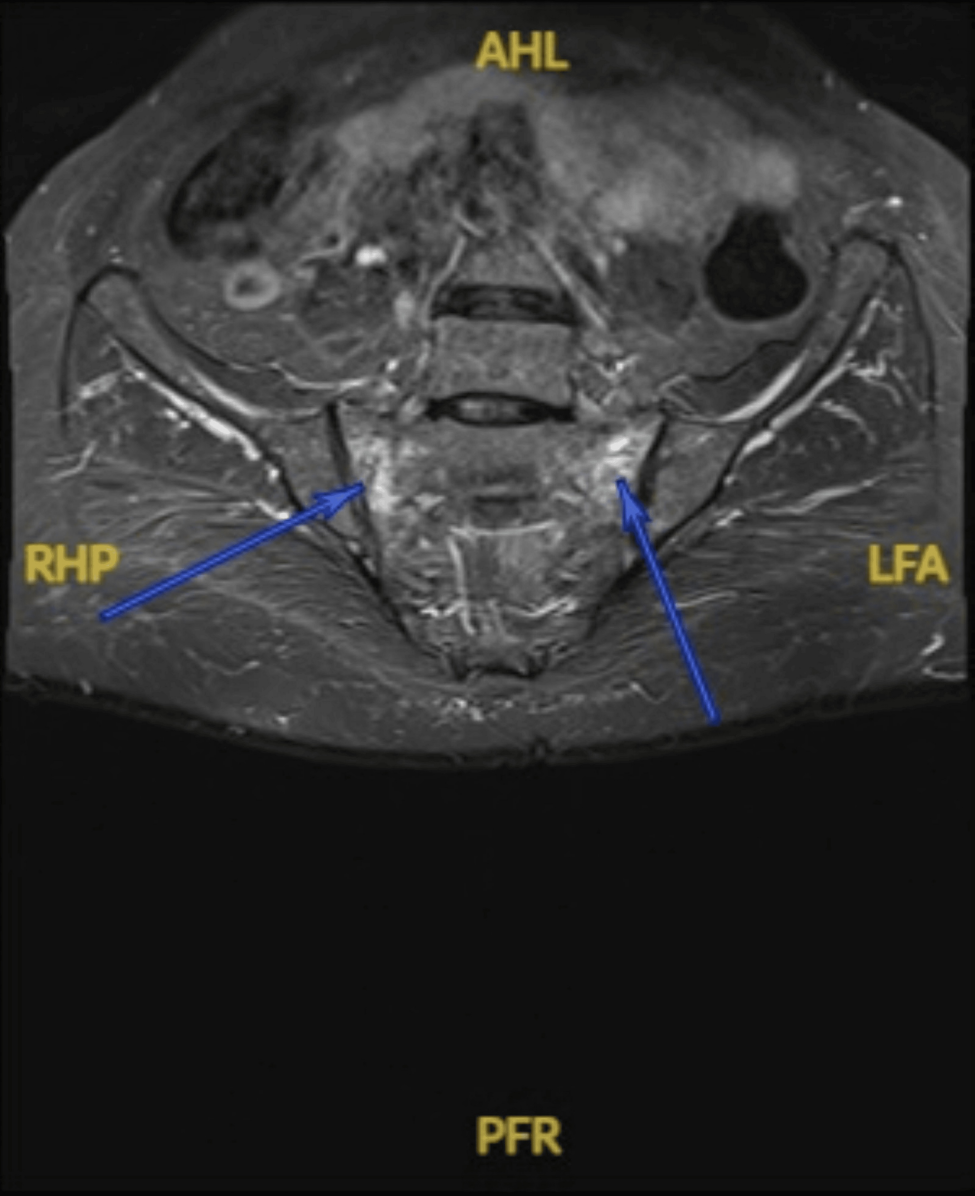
MRI of the sacrum, T2-weighted coronal images, showing bilateral sacral ala insufficiency fracture mostly in zone 1 between S1 and S3. AHL, anterior high left; RHP, right high posterior; LFA, left fossa anterior; PFR, posterior fossa right
